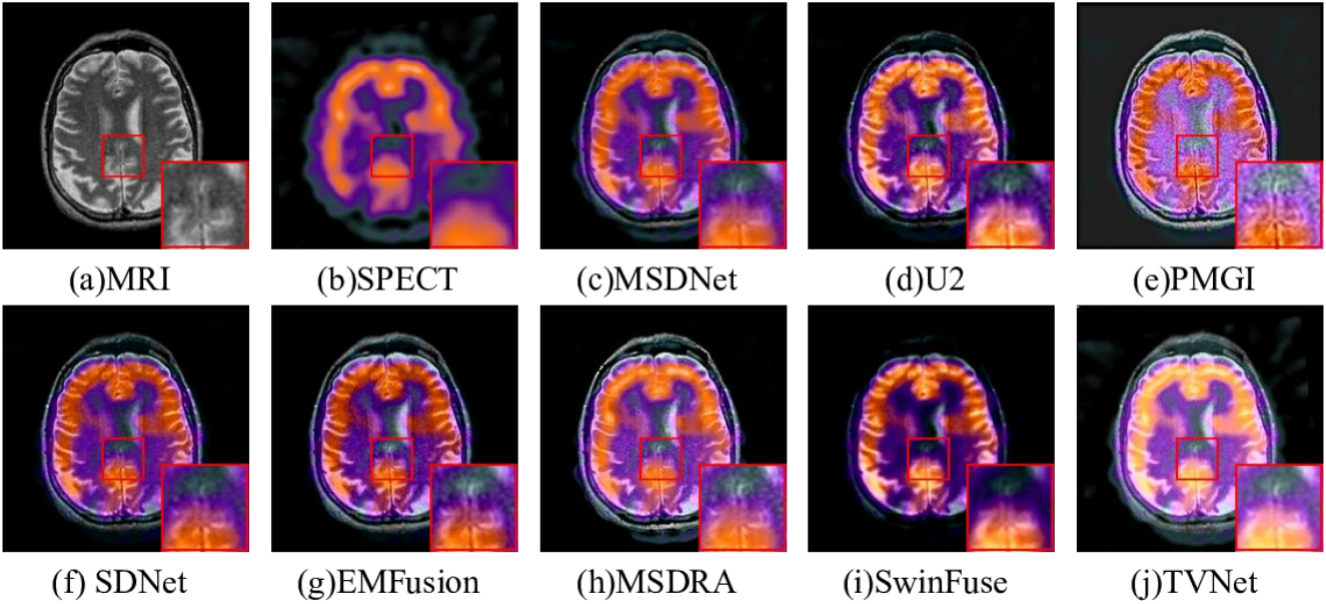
Comparison on single-photon emission computed tomography-magnetic resonance imaging (SPECT-MRI) fusion.
